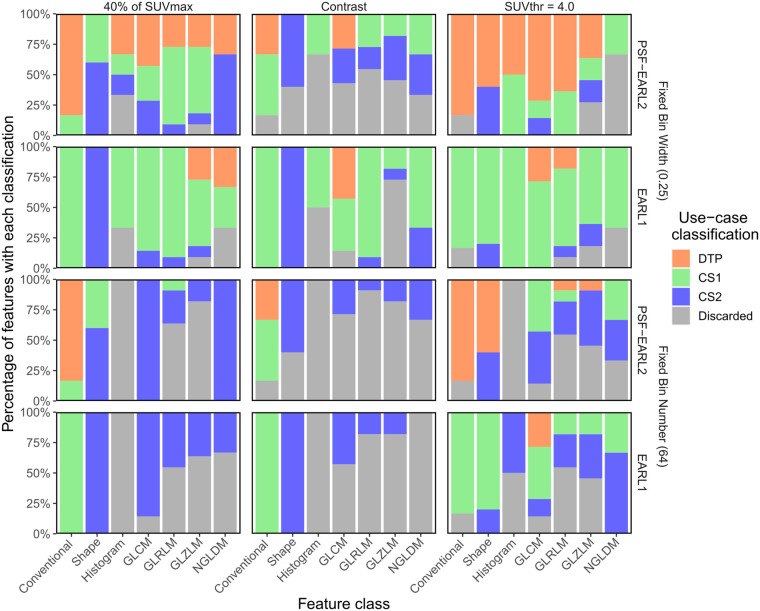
Percentage of radiomic features with each use-case classification for each feature class in all image setting configurations. Columns of panels show different lesion delineation methods, and rows show different image reconstructions and intensity discretization strategies. Analysis was of images with reference settings. DTP = dual time point; SUVthr = SUV threshold.
